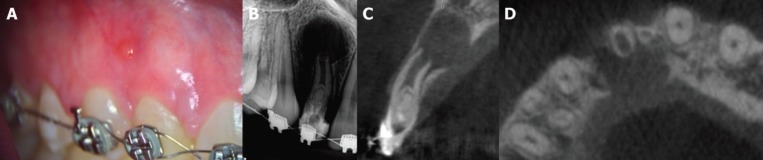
A. Clinical examination showing a sinus tract. B. Radiographic examination showing a large periradicular radiolucency, an open apex and a complex canal anatomy with type II DI. C. Sagittal and D. axial CBCT images showed the invagination extending beyond the cementoenamel junction, reaching the pulpal space and periapical radiolucency disrupting the bone cortical palatal.
